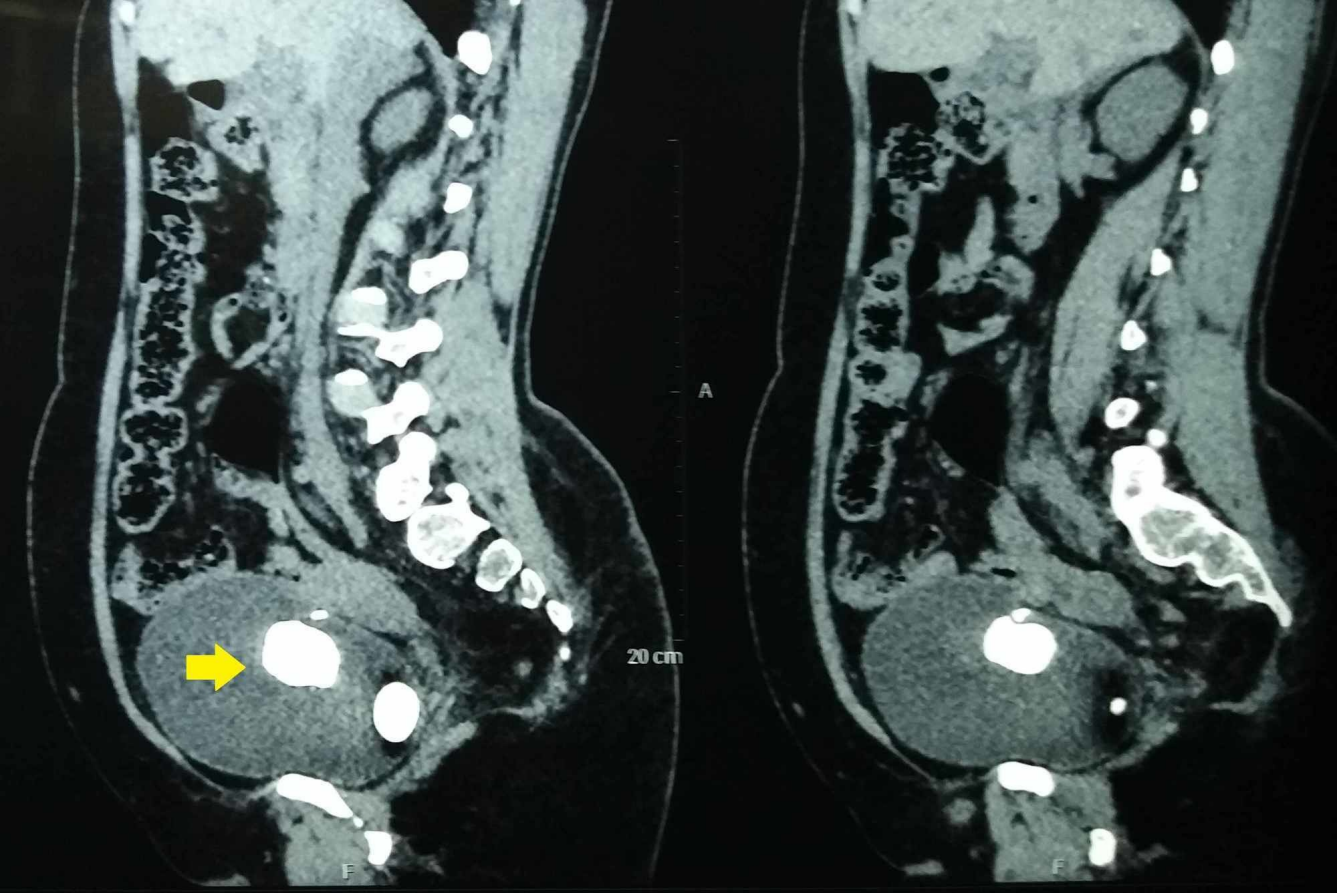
Sagittal section of noncontrast computed tomography depicting two bladder stones, with one hanging from the bladder wall partly embedded within bladder mucosa (yellow arrow)
